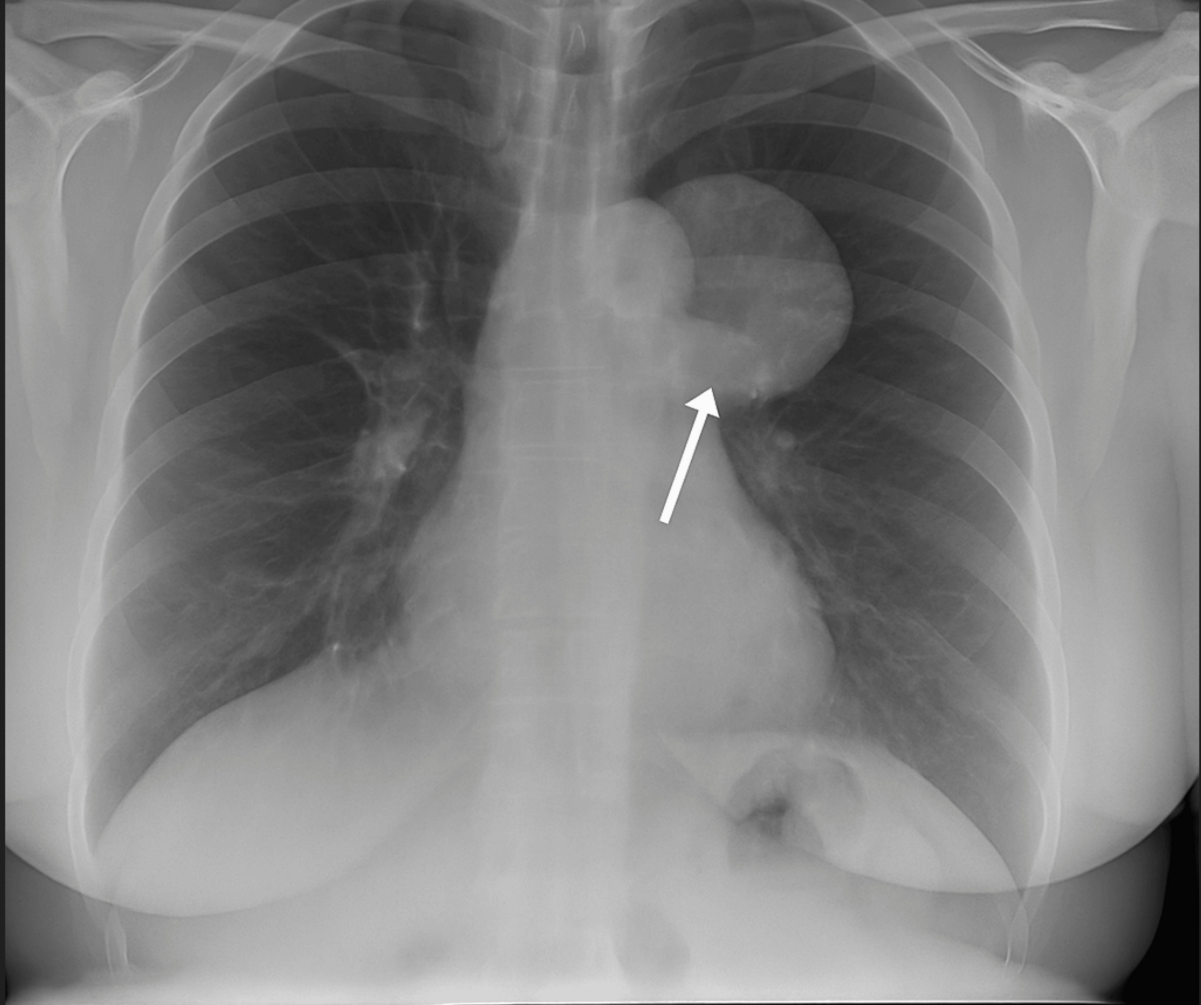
Chest radiograph (posteroanterior view) showing a well-defined, rounded opacity in the left upper mediastinal region.
